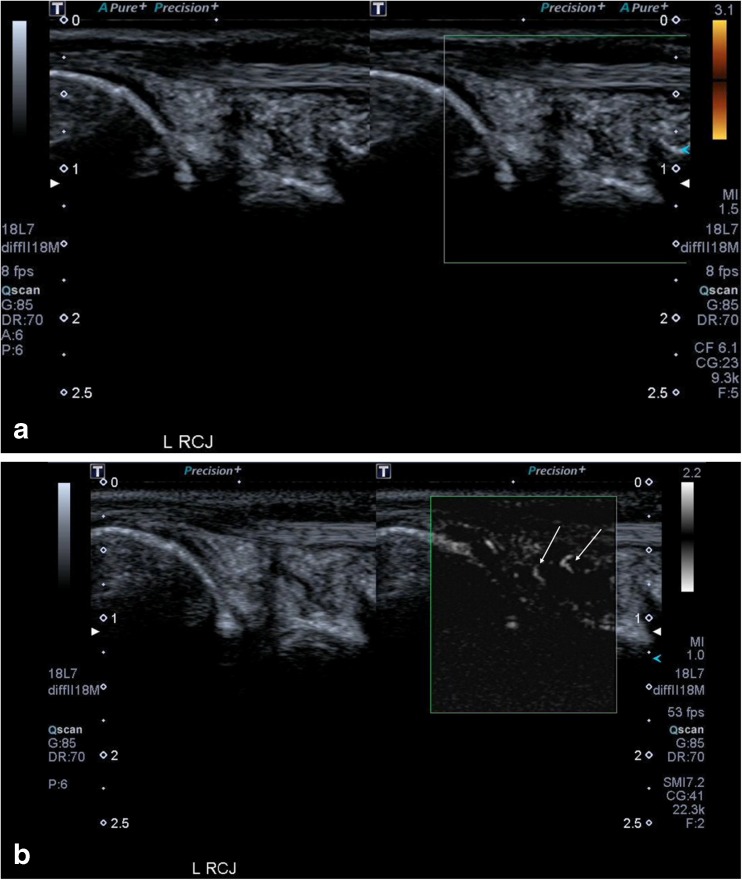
(a) These images show synovial hypertrophy on the grey-scale image of the radiocarpal joint (RCJ) in a patient with rheumatoid arthritis. In the dual image the right side of the split screen shows no detectable vascular flow within the thickened synovium on Power Doppler ultrasound (PDUS). (b) This second pair of images shows that there is clear neovascularity within the joint (arrows) on Superb Microvascular Imaging (SMI) indicating active inflammation, which was not evident on PDUS. The increased sensitivity and spatial resolution of SMI is much better appreciated on videoclips rather than still images and these have been included as supplementary material for this patient
